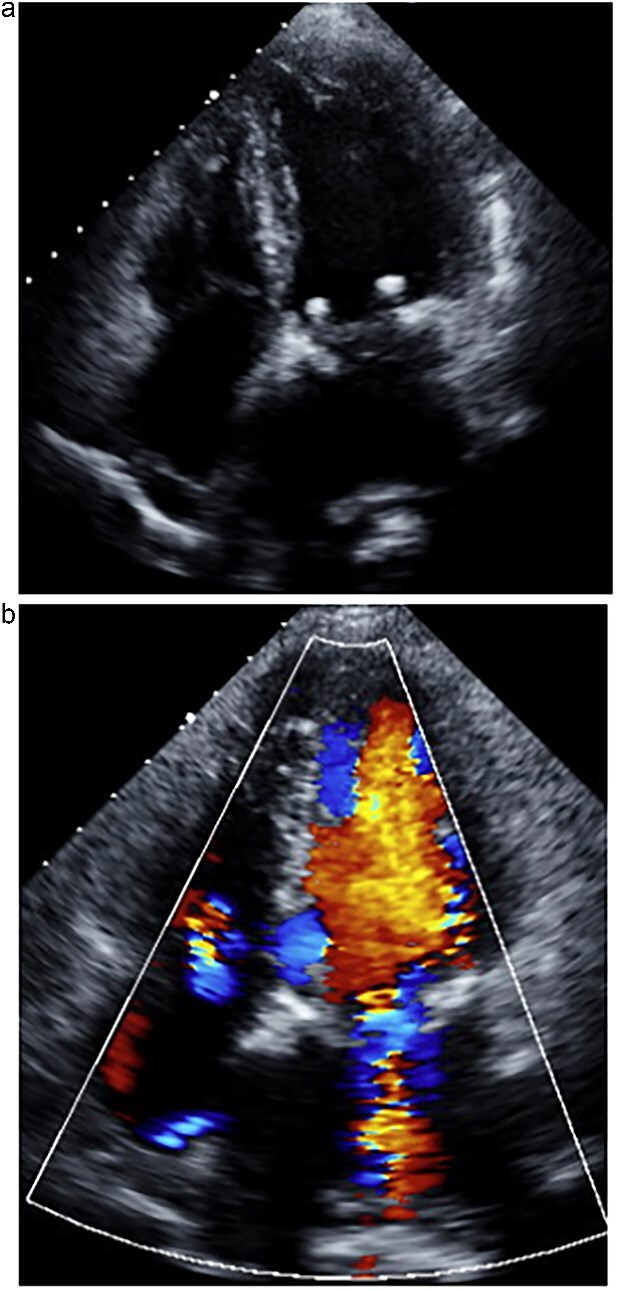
One week postoperative transthoracic echocardiography. (a) The left atrium was reduced in size. (b) Mitral regurgitation was well controlled.
